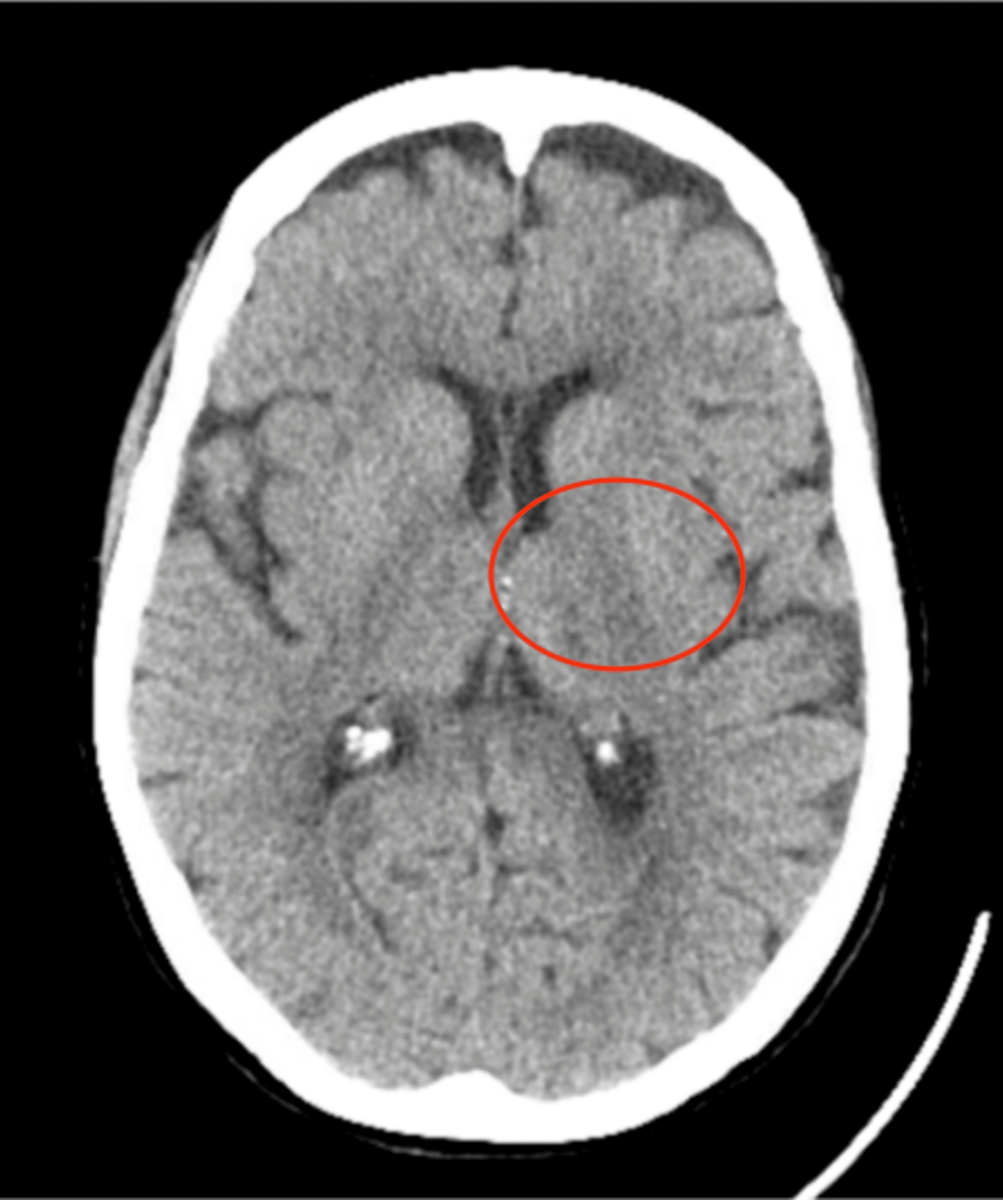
Head CT showing remote lacunar infarct in the left thalamus (circled in red) CT, computed tomography
